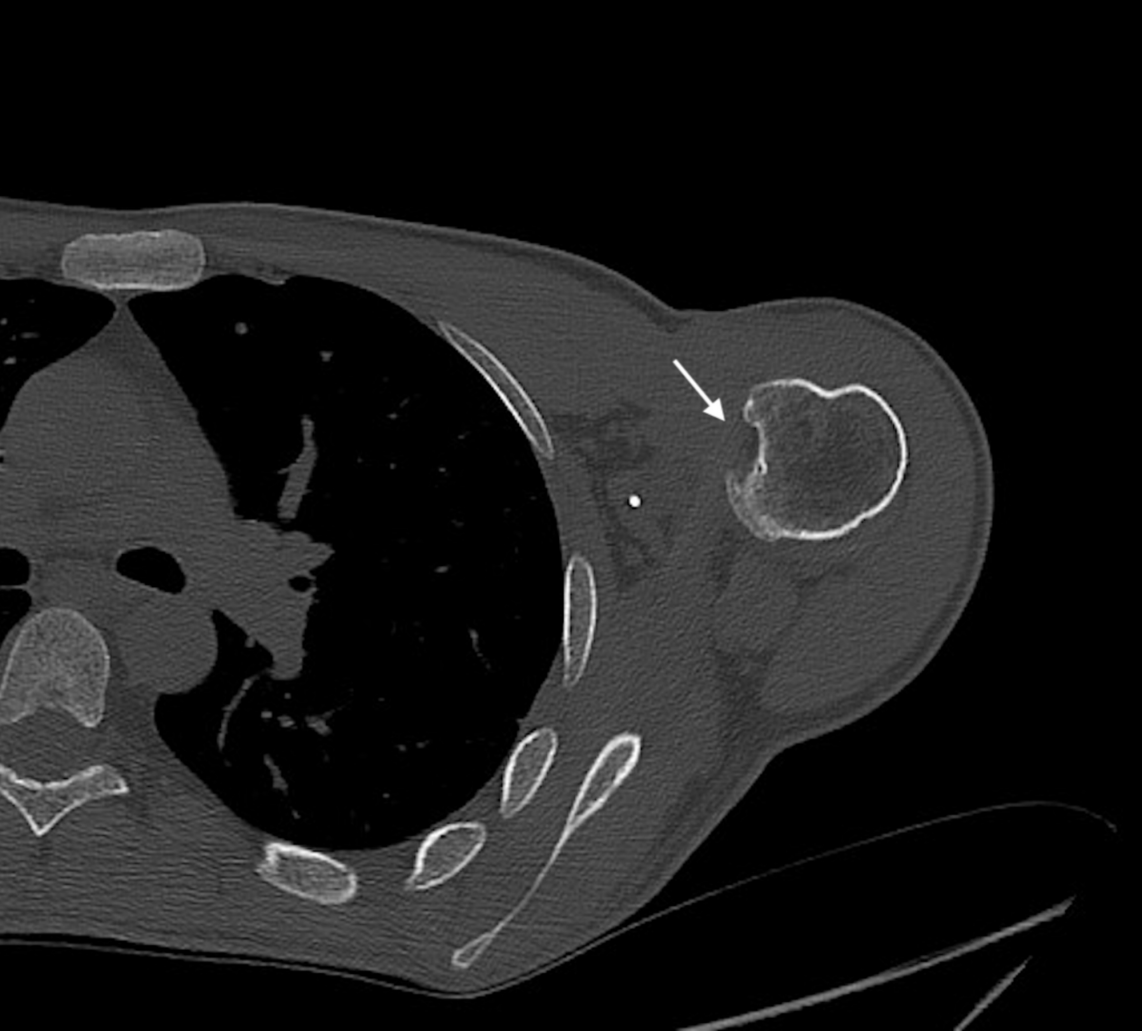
Axial CT shoulder showing a large reverse Hill-Sachs deformity CT: computed tomography
